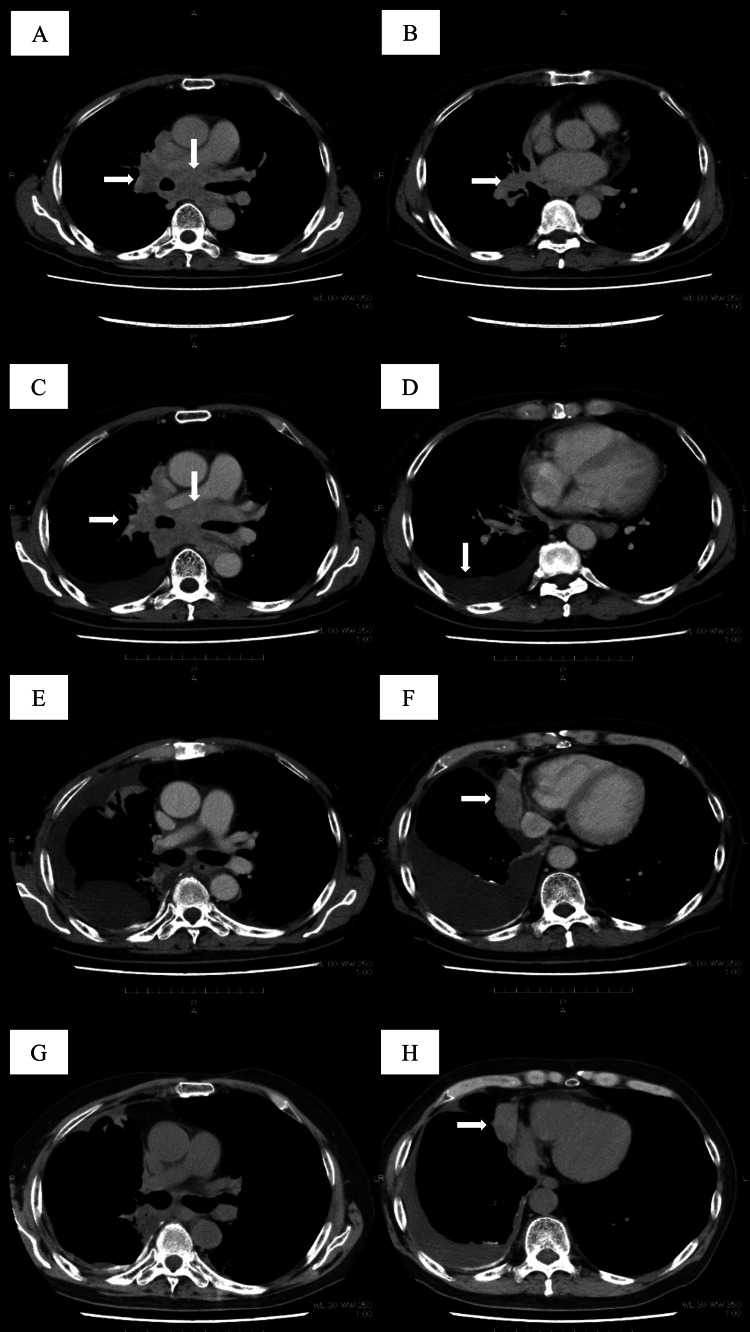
CT scan findings during the patient's treatment. Areas of interest described are demonstrated by the white arrows. (A)(B) Computed tomography (CT) scan findings before the first line of chemotherapy demonstrate findings of mediastinal lymph node enlargement and a right lower lobe tumor. (C)(D) Six months after initiation of his first-line chemotherapy, a newly diagnosed pleural effusion was revealed, while his mediastinal lymph nodes and right lower lobe tumor remained stable. (E)(F) Prior to the administration of his third-line amrubicin treatment, his previous therapy with palliative irradiation, four cycles of nogitecan, and pleurodesis result in fair control of the right pleural effusion. However, a newly found tumor in his right lung adjacent to the heart is revealed. (G)(H) Imaging studies reveal stable findings 16 months following the initiation of his third-line treatment with amrubicin.
